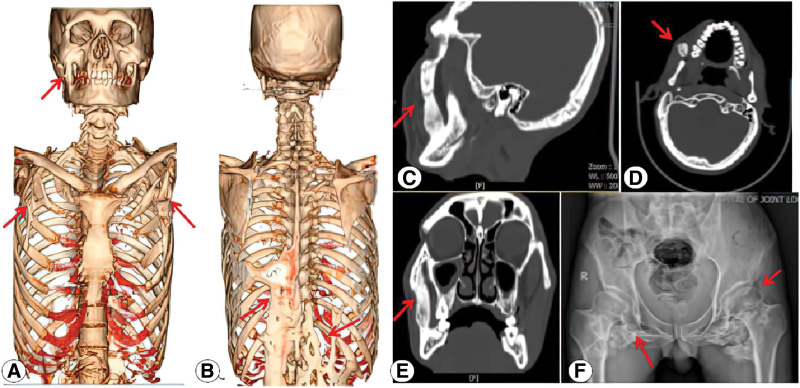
Three-dimensional reconstruction image of head, chest, and abdomen and X-ray image of the pelvis. (A) Ectopic ossification in masseter, scapular region; (B) Bony connections in the lower part of the spine; (C) sagittal view of ectopic ossification in masseter; (D) axial view of ectopic ossification in masseter; (E) coronal view of ectopic ossification in masseter; (F) high-density shadow around the bilateral femur and ilium.
